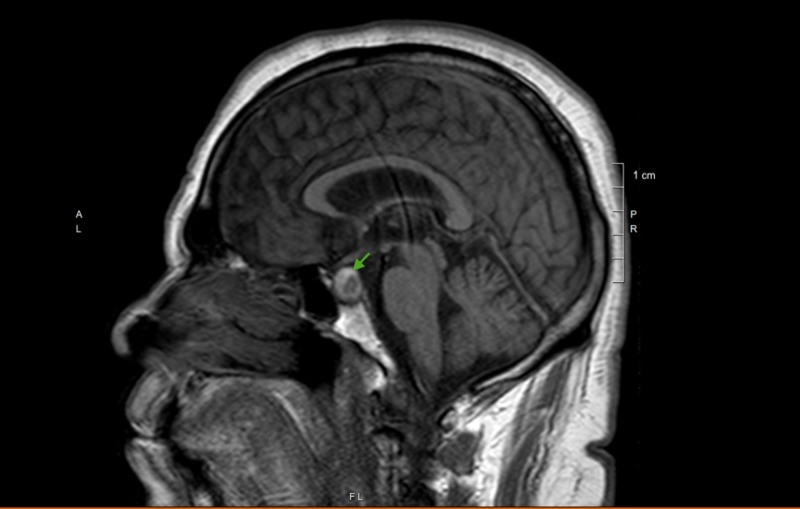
T1 sagittal section of magnetic resonance imaging of the brain showing pituitary mass with peripheral hyperintensity
